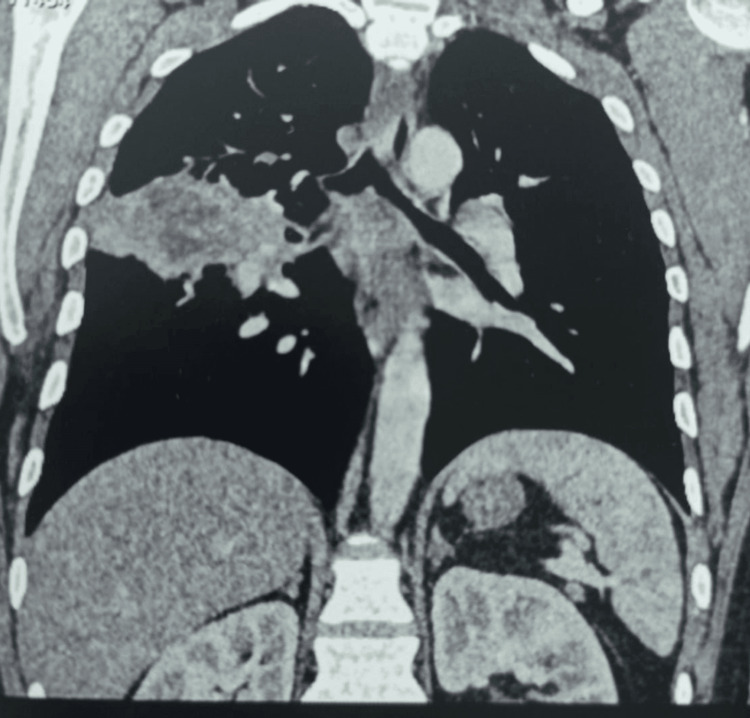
Chest computed tomography confirmed a cavitary consolidation
